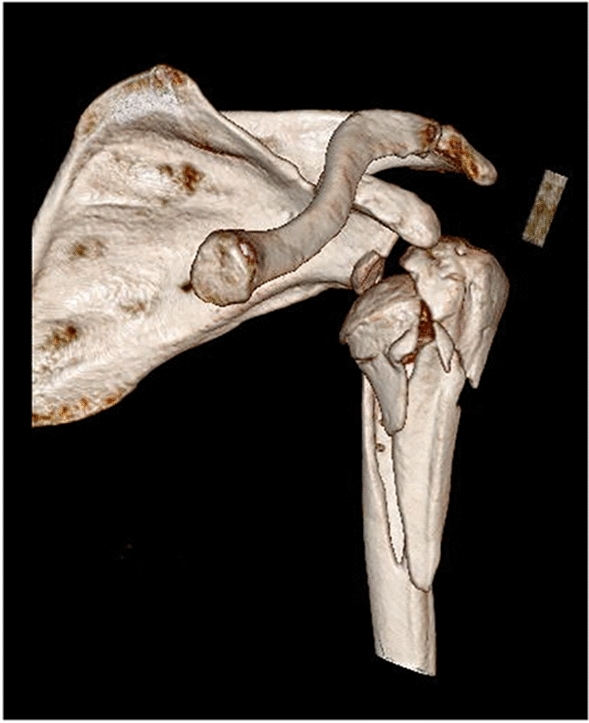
CT three-dimensional reconstructed coronal image of a right sided comminuted proximal humeral and glenoid fracture
